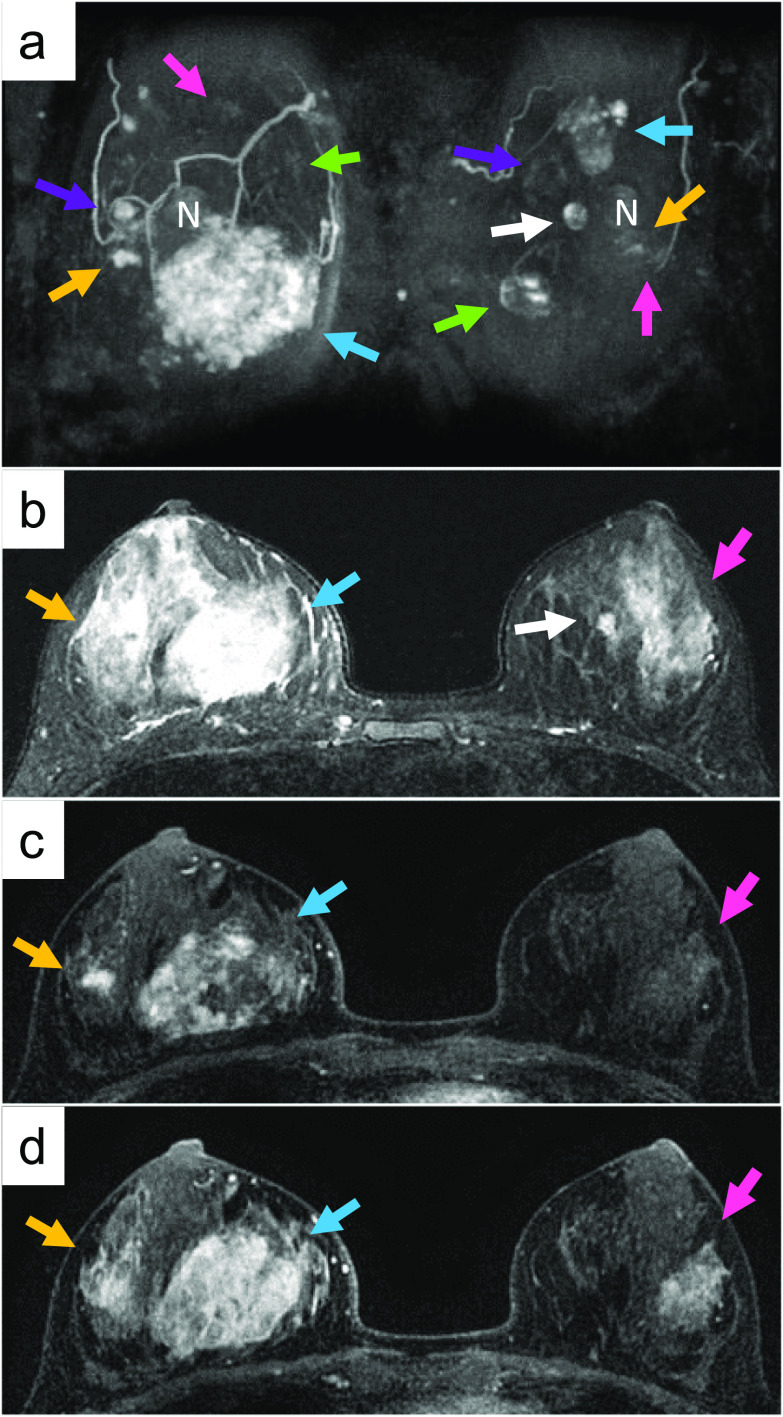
Magnetic resonance imaging showed five heterogeneously enhanced masses, 5.8-cm-diameter at a maximum, in the right breast, and six enhanced masses, approximately 1–3 cm in diameter, in the left breast (arrows in a). These masses showed high intensity on fat-suppressed T2-weighted images (b). The contrast-enhanced images exhibited heterogeneous enhancement in the early phase (c), and progressive and concentric enhancement in the delayed phase (d). N: nipple
